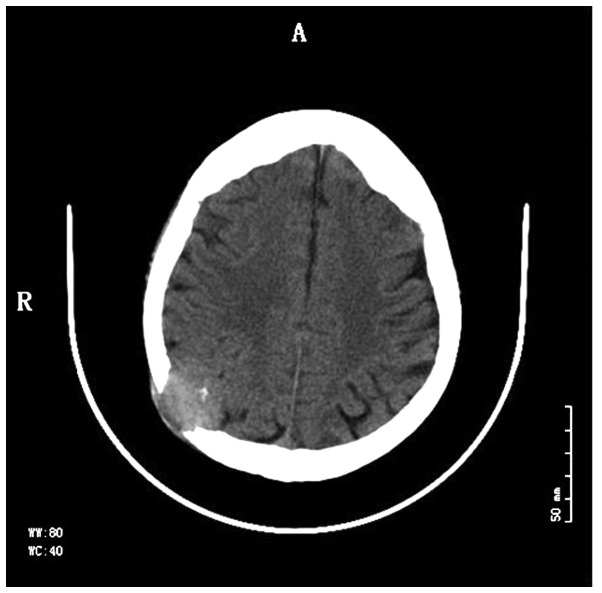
Case 2. Mass destruction and skull defect in the right parietal bone by computed tomography.
